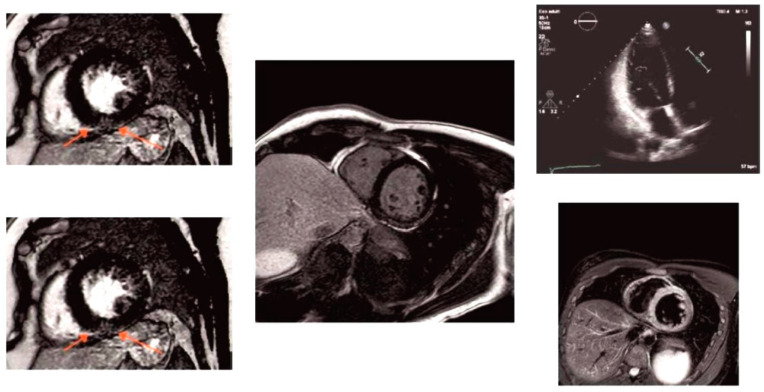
Cardiac magnetic resonance (MRI) showed late gadolinium enhancement of the inferior junctional and inferior wall in the middle segment (red arrows).
